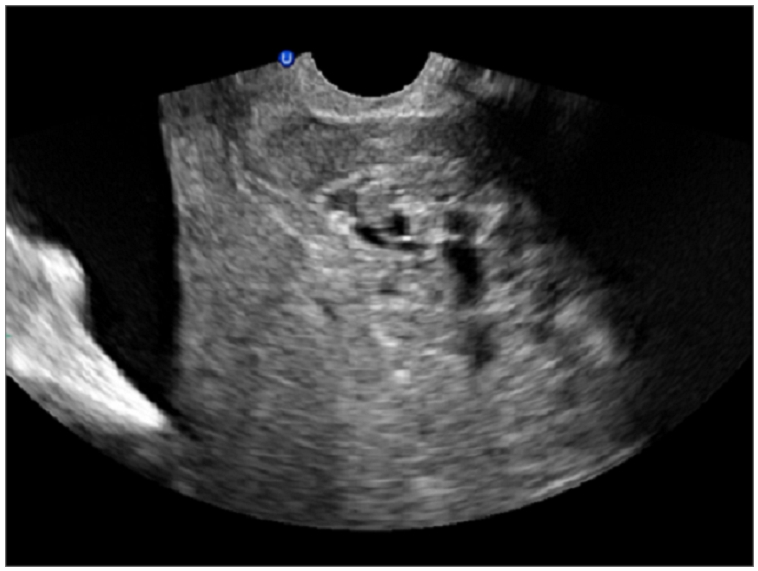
transvaginal ultrasonography showing a heterogeneous mass in the uterine cavity in a patient with Ovarian hyperstimulation syndrome in a spontaneous pregnancy with invasive mole
